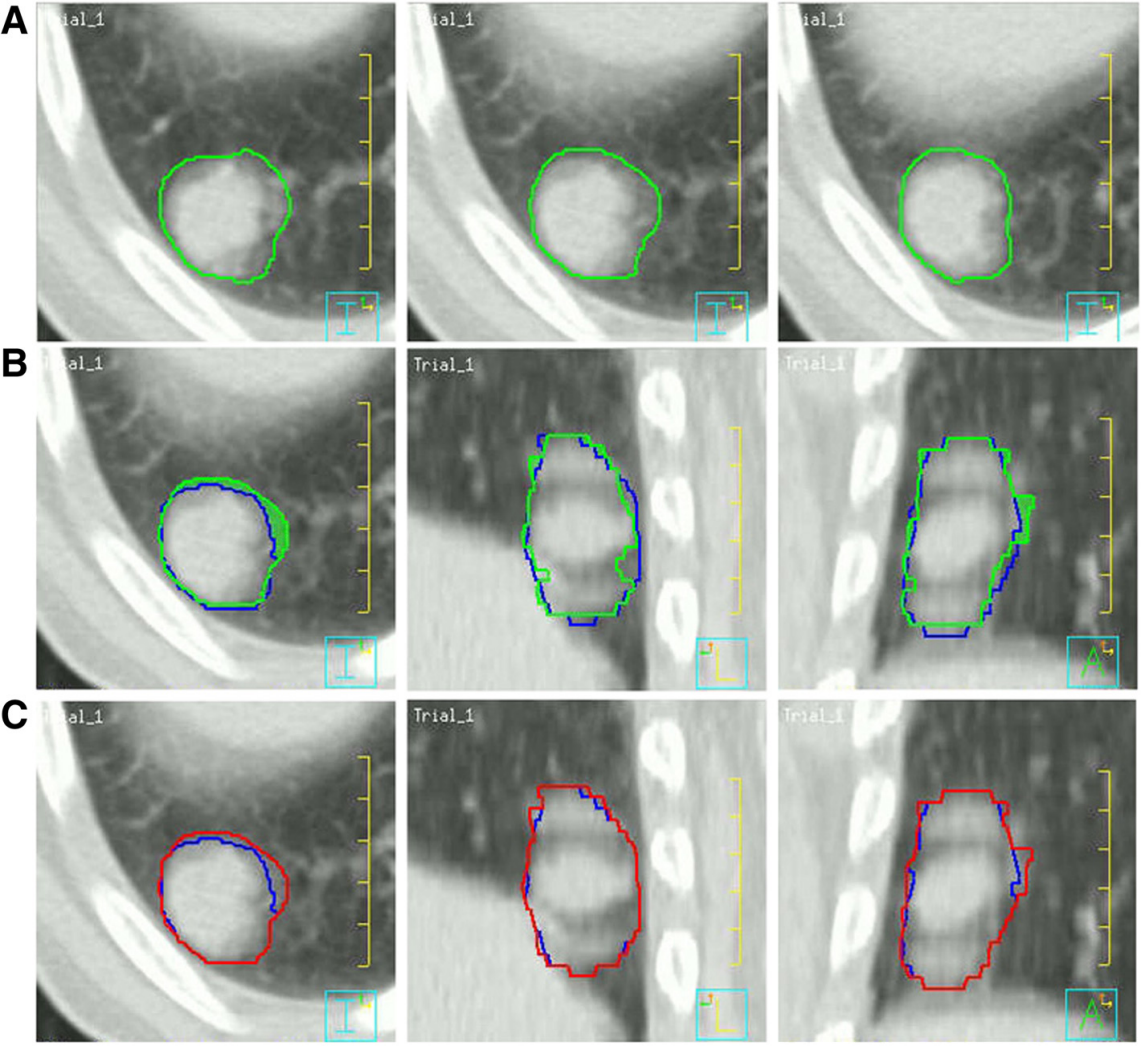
Example of different ITVs in a lung cancer patient (tumor No. 3). Transverse, sagittal, and coronal views of ITVslow (green), ITVall (blue), and ITVall+slow (red) are projected onto a modified slow CT scan. A. Delineation of ITVslow. B. Relationship between ITVall and ITVslow. The geometric regions of ITVslow that are missed by ITVall are displayed as green colored regions. C. Relationship between ITVall and ITVall+slow.
